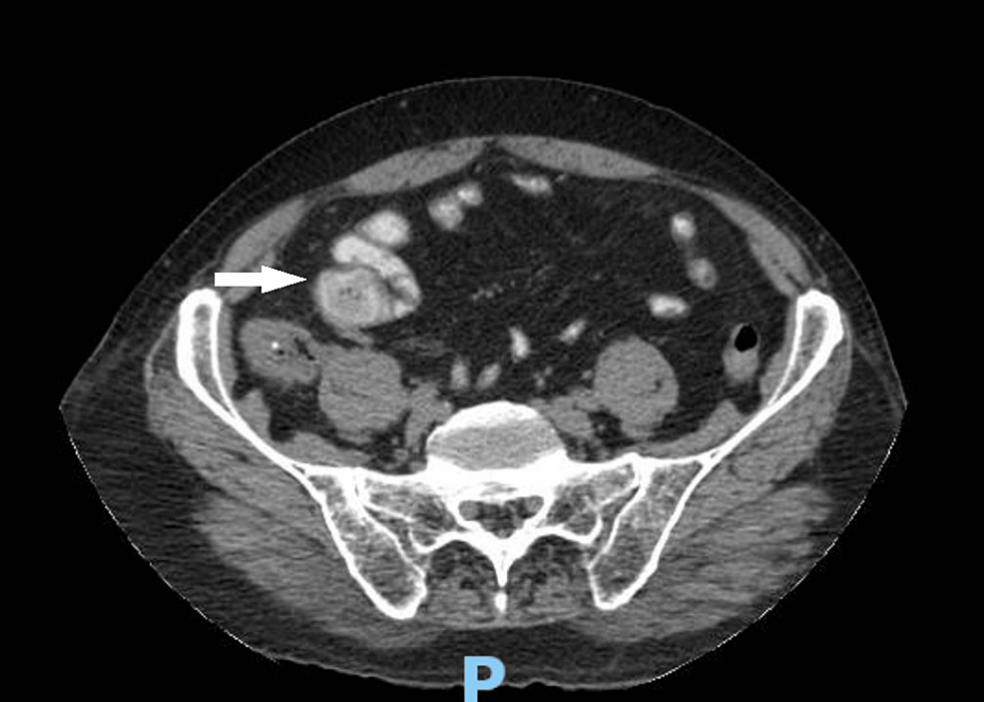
CT abdomen The arrow shows the Mercedes Benz sign with the proximal bowel loop dilated and collapsed distal loops.
